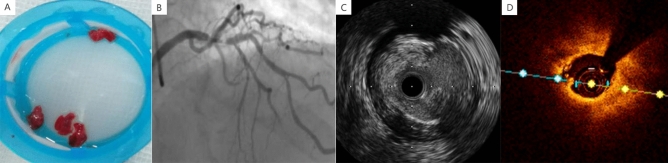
Four different states of thrombus, which were labeled as (A–D) in turn: (A) In vitro; (B) Coronary angiography; (C) Intravascular ultrasound; (D) Optical tomography.
